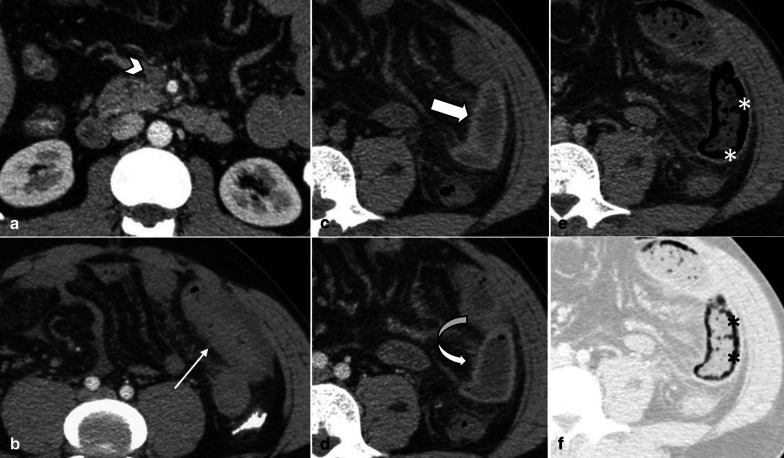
A 32 y/o male presenting with acute SMV thrombosis due to protein C deficiency. CT images on first day of admission (a, b) display thrombosis (arrowhead) in SMV with segmental circumferential mural thickening in ileum (thin arrow), mesenteric fat stranding and free fluid. On day 3 (c, d), mural hyperdensity (thick arrow) is noted in non-contrast CT at the same segment without post contrast hyper-enhancement (curved arrow) suggestive of intramural hemorrhage. Mural thickening has relatively decreased. On day 9 (e, f), mural pneumatosis (asterisks) and thinning (paper thin) are depicted consistent with development of transmural necrosis which was confirmed in laparotomy
